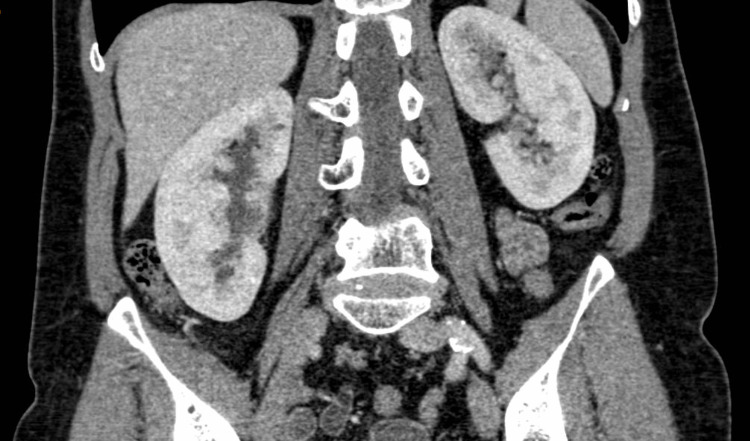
Two months follow-up CT scan. Sagittal section showing absence of pyelocaliceal dilatation.
